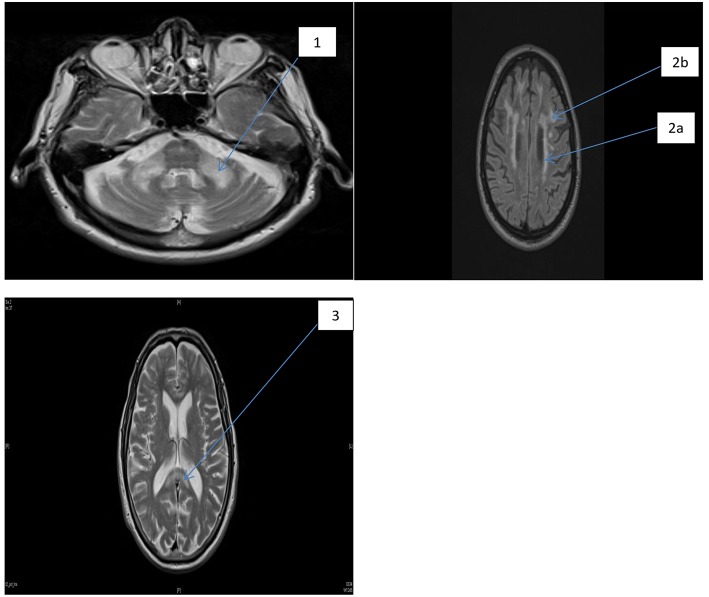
FLAIR MRI axial section of two representative patients with FXTAS. MCP sign and foci of *wmhs* (1), the periventricular *wmhs* (2a), deep *wmhs* (2b), and *wmhs* in the splenium of the corpus callosum (3).
